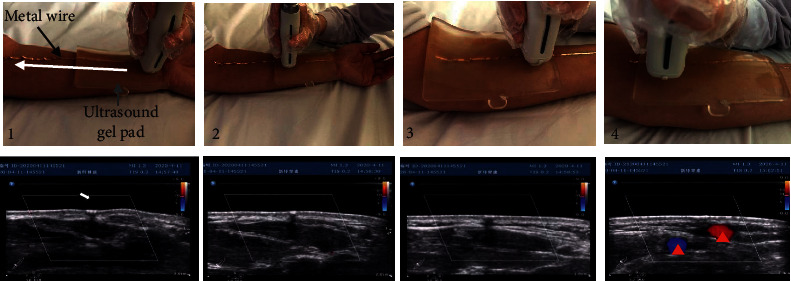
Transverse ultrasound imaging of fluorescent line. As shown in upper images, metal wire was overlaid on the fluorescent line and fixed in place with a rectangular ultrasound gel pad. Transverse images were obtained as the ultrasound probe followed the course of the metallic wire from PC6 toward PC3. The metal wire is seen as an echogenic white dot (white arrow in left lower picture), casting a B-scan shadow. Color Doppler signal was only seen at the end of scanning (near elbow PC3) but not directly under the metallic wire.
